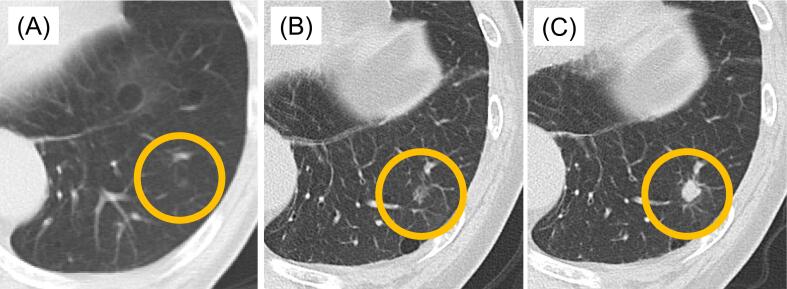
(A) Chest CT images showing a small (4-mm) ground glass opacity. (B—C) The small ground glass opacity was gradually enlarged over two years and six months (B), becoming a small (9-mm) solid nodule with spiculation and lobulation after three years (C).
